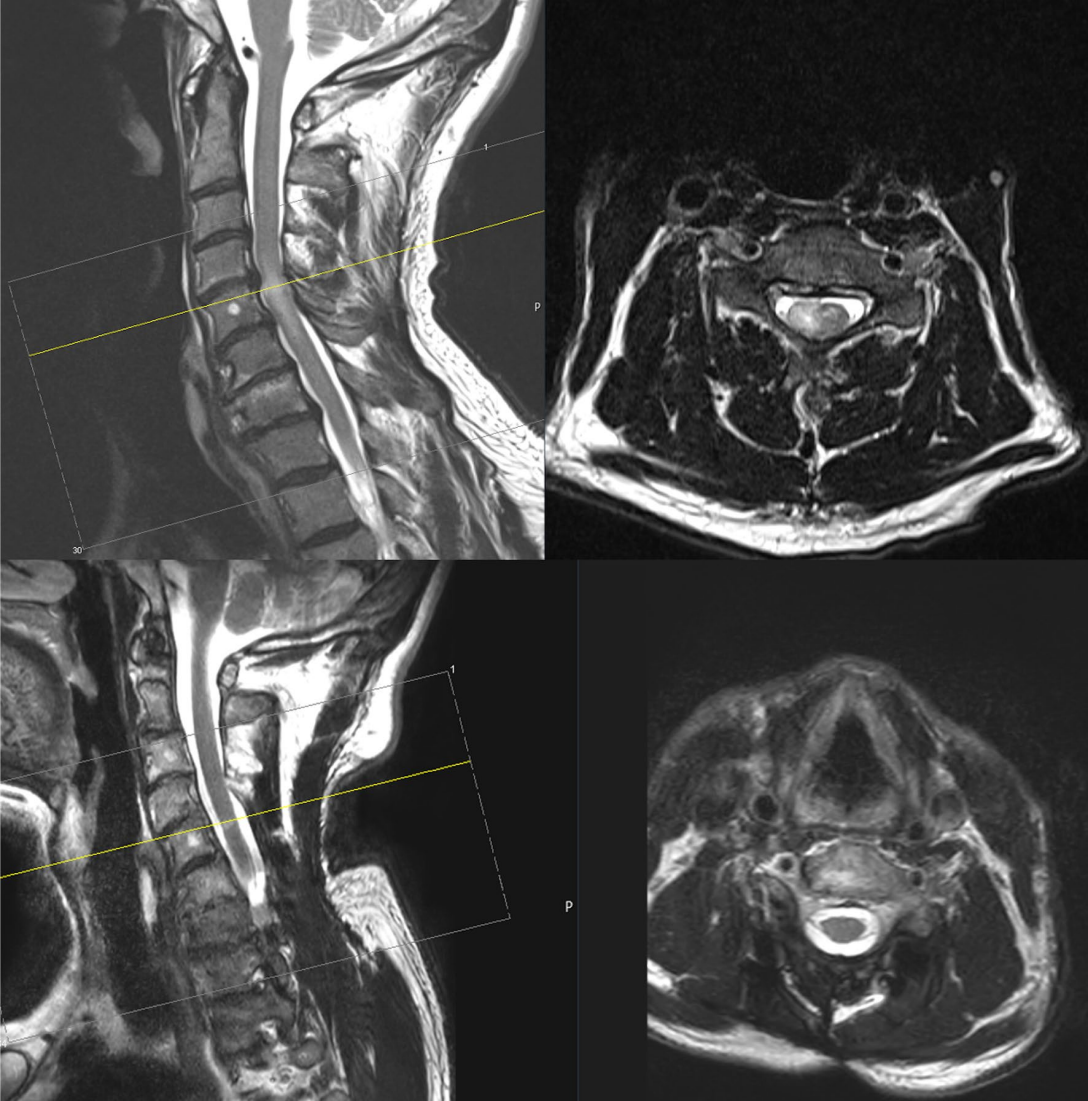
Preoperative (upper image) T2 sequenced MRI of a patient suffering from an intramedullary diffuse astrocytoma WHO II C4–6 with complete tumor resection and MRI imaging after 7 years (lower image) without tumor recurrence
